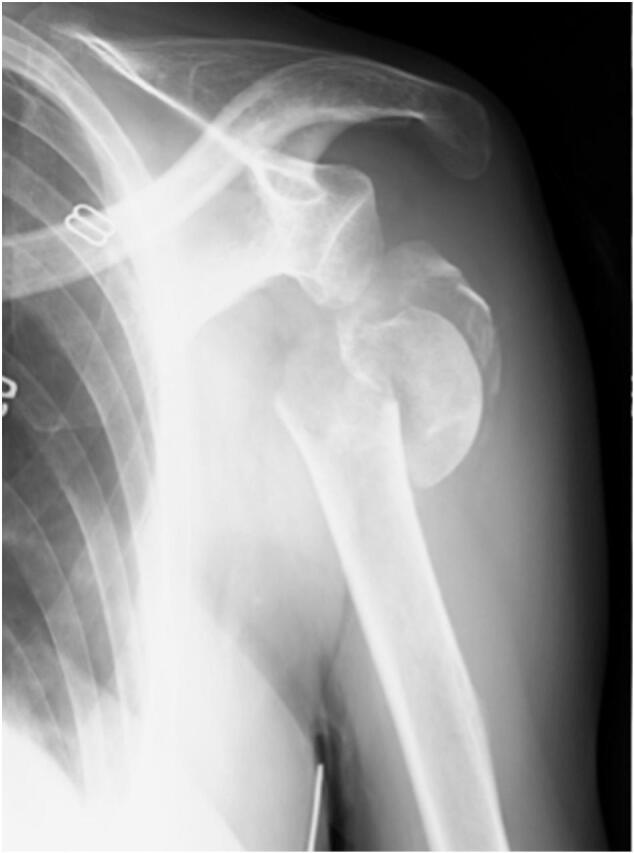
A plain radiograph of the left shoulder shows a four-part fracture dislocation, with the distal fragment displaced medially.
